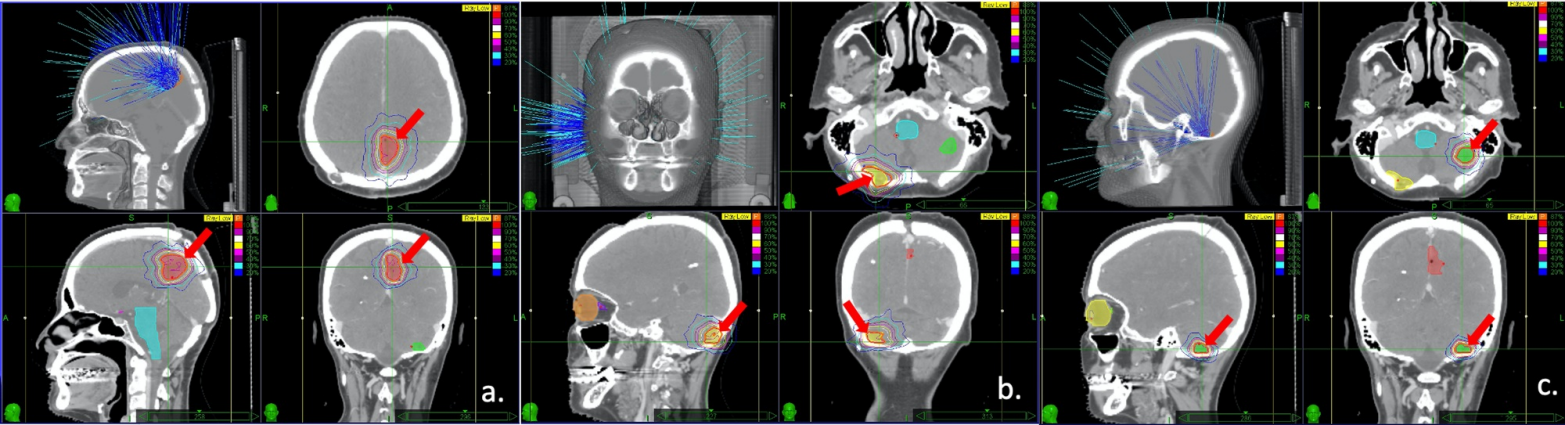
Treatment planning images a. Axial, sagittal, and coronal views of the treatment planning computed tomography (CT) scan of the resection cavity in the left parietal lobe. The orange line is the prescribed isodose (87%). b. Axial, sagittal, and coronal views of the treatment planning CT scan of the lesion in the right cerebellar hemisphere. The orange line is the prescribed isodose (88%). c. Axial, sagittal, and coronal views of the treatment planning CT scan of the lesion in the left cerebellar hemisphere. The orange line is the prescribed isodose (87%).
